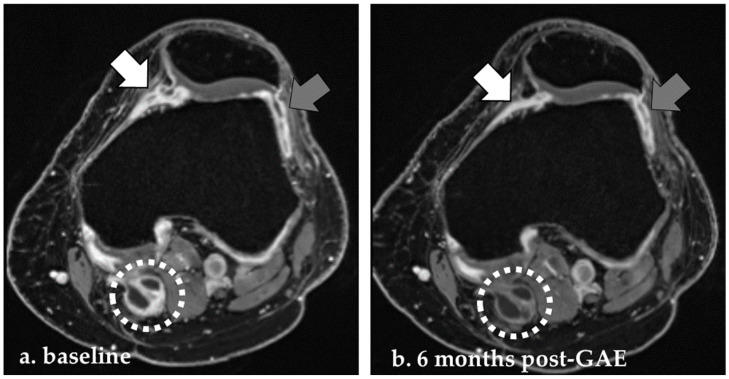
CE-MRI at baseline and 6 months post-GAE. On axial CE-MRI, the synovial thickness of the medial (white arrow) and lateral parapatellar (grey arrow) areas, as well as a possible Baker’s cyst (dotted circle), were included in the whole-joint synovitis score along with areas scored at sagittal images. This patient, treated on the medial side of the left knee, showed a reduction in both local and whole-joint synovitis scores 6 months post-GAE (b) compared to baseline (a).
